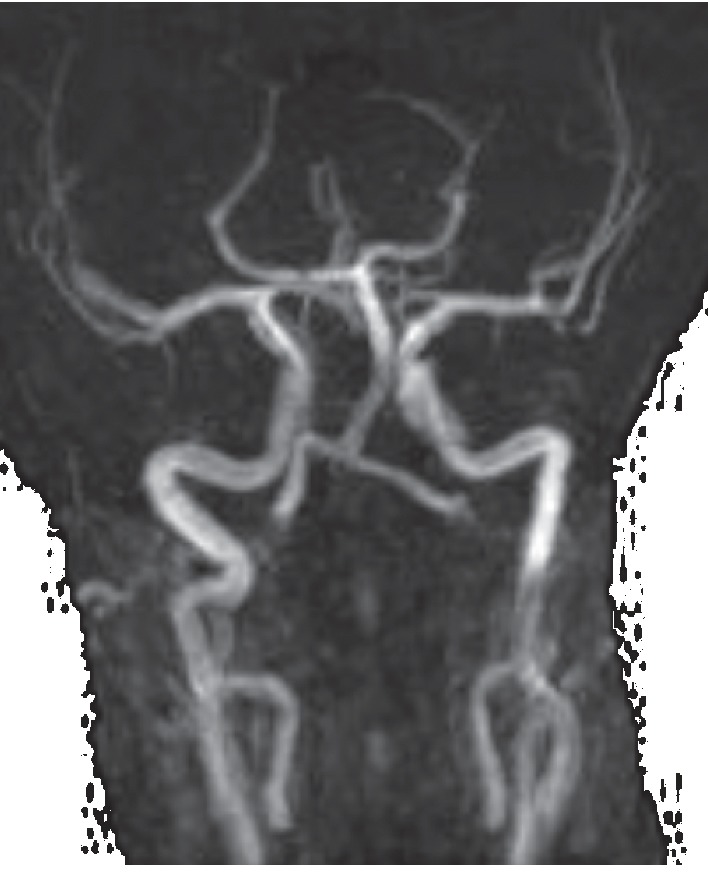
TOF 3D MRI angiography 10 days after treatment reveals total recalibration of the internal carotid artery.
